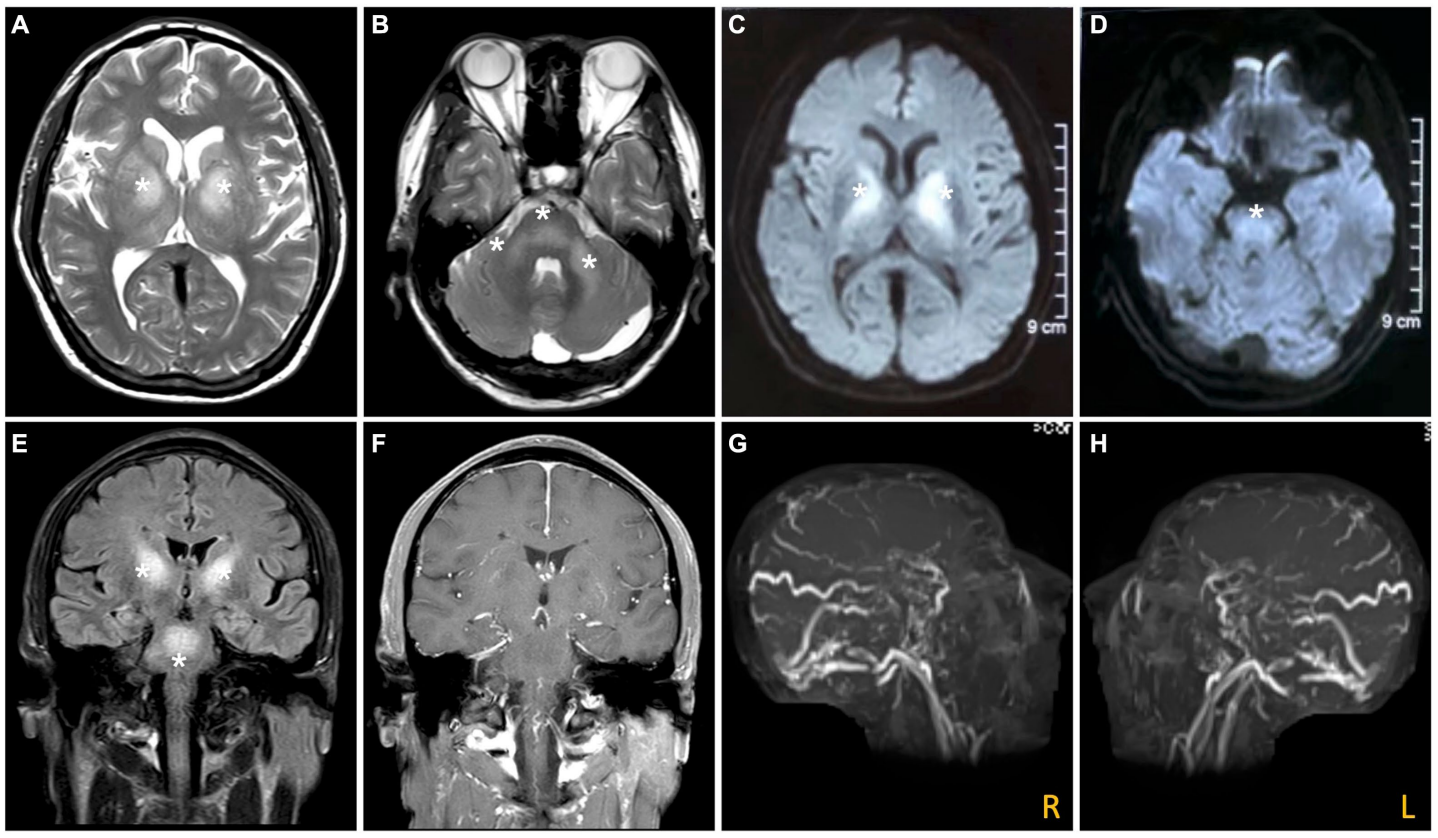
Brain MRI and MRV. The axial section of the T2-weighted image (A,B) and the diffusion-weighted image (C,D) reveal bilateral symmetrical hyper-intensities in the basal ganglia and brain stem (white asterisk). The lesions present with hyperintensity on fluid attenuated inversion recovery (FLAIR) images (E), and no enhancement on enhancing MRI (F). The MRV (G,H) indicates a filling defect in the right transverse, left sigmoid, and superior sagittal sinuses.
